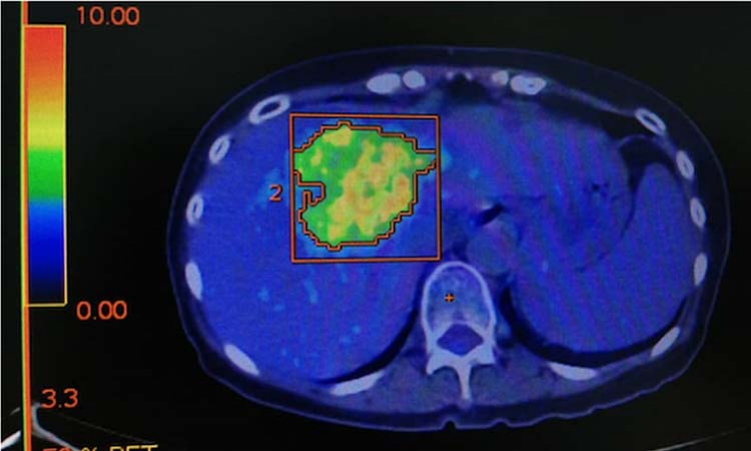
PET CT demonstrating residual liver tumour after 8 cycles of chemotherapy.
